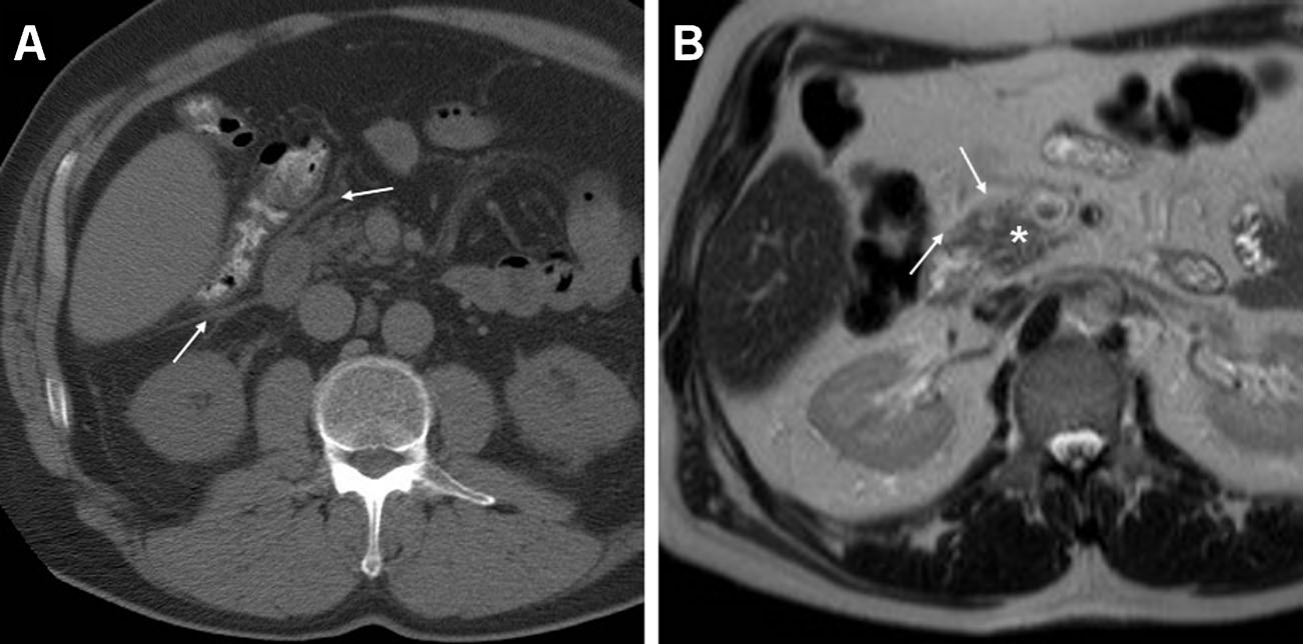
Oedematous AP with markedly increased serum lipase 48 h after ERCP treatment of choledocholithiasis in a 55-year-old male with previous history of biliary AP and laparoscopic cholecystectomy. Unenhanced CT (a) and T2-weighted MRI (b) images show mild peripancreatic and retroperitoneal fascial fluid (*thin arrows*) without abnormal collections. Clinical symptoms and laboratory changes regressed within 72 h
